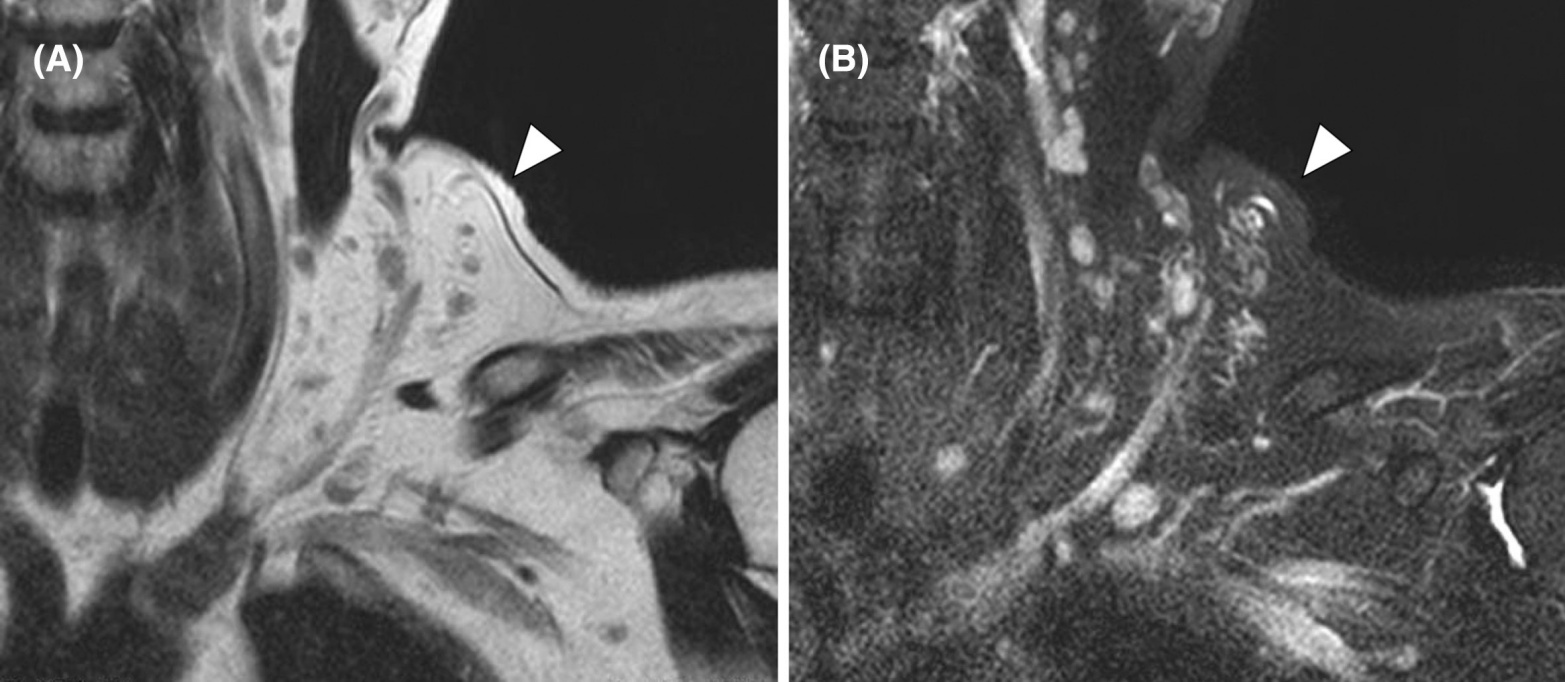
Coronal contrast‐enhanced magnetic resonance images. The lesion (arrowhead) had a borderline indistinct margin with (A) signal intensity equal to that of fat on T1‐weighted imaging and (B) high signal intensity on fat‐suppressed T2‐weighted imaging.
